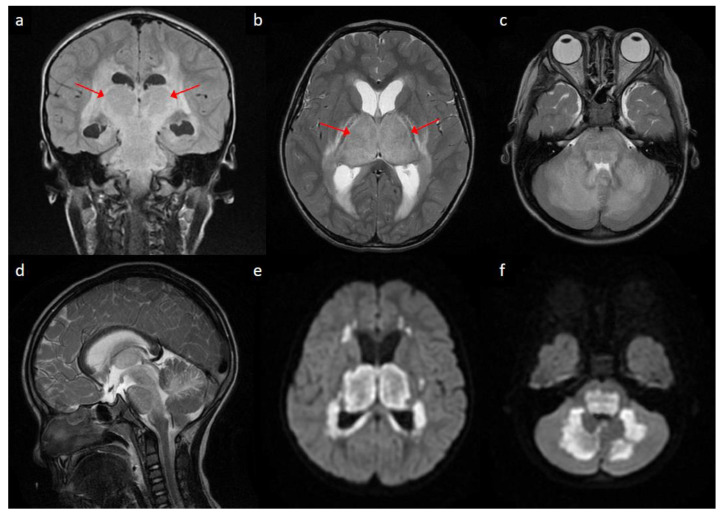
Brain magnetic resonance imaging of the patient: (a) coronal view of T2 fluid-attenuated inversion recovery sequence showing hyperintense signals in bilateral thalami (arrows) and periventricular regions; (b,c) Axial view of T2-weighted sequences at the level of basal ganglia/thalami (arrows) and pons/cerebellum, respectively, showing relatively symmetric hyperintense signals in bilateral thalamic, periventricular, pontine and cerebellar regions; (d) sagittal view of T2-weighted sequence showing hyperintense signal changes in brainstem and cerebellum; (e,f) axial view of diffusion-weighted imaging at the levels corresponding to (b,c), respectively, showing bright signals in bilateral thalamic, periventricular, pontine and cerebellar regions.
